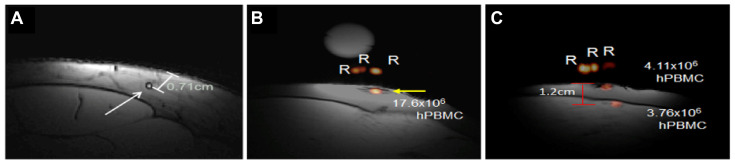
MRI detection of ^19^F-labeled human PBMCs in a clinically relevant model at 3T. (A) Representative proton image of a human leg obtained with the same orientation and imaging parameters demonstrates tissue appearance and subcutaneous fat deposition similar to those found in the ham shank model shown in (B,C); the arrow marks a lymph node which serves as injection depth calibration for the ham shank, located at an approximate depth of 0.71 cm. (B) ^19^F MRI demonstrates detectable signal following intradermal injection of 17.6 × 10^6^ labeled human PBMC in the ham shank. (C) ^19^F MRI also detects lower administered cell doses, including 4.11 × 10^6^ labeled human PBMCs injected intradermally and 3.76 × 10^6^ labeled human PBMCs injected intramuscularly, with the deeper signal visualized at approximately 1.2 cm below the surface. R, reference markers. PBMCs, peripheral blood mononuclear cells. Adapted from [134] under a Creative Commons Attribution 4.0 International License.
